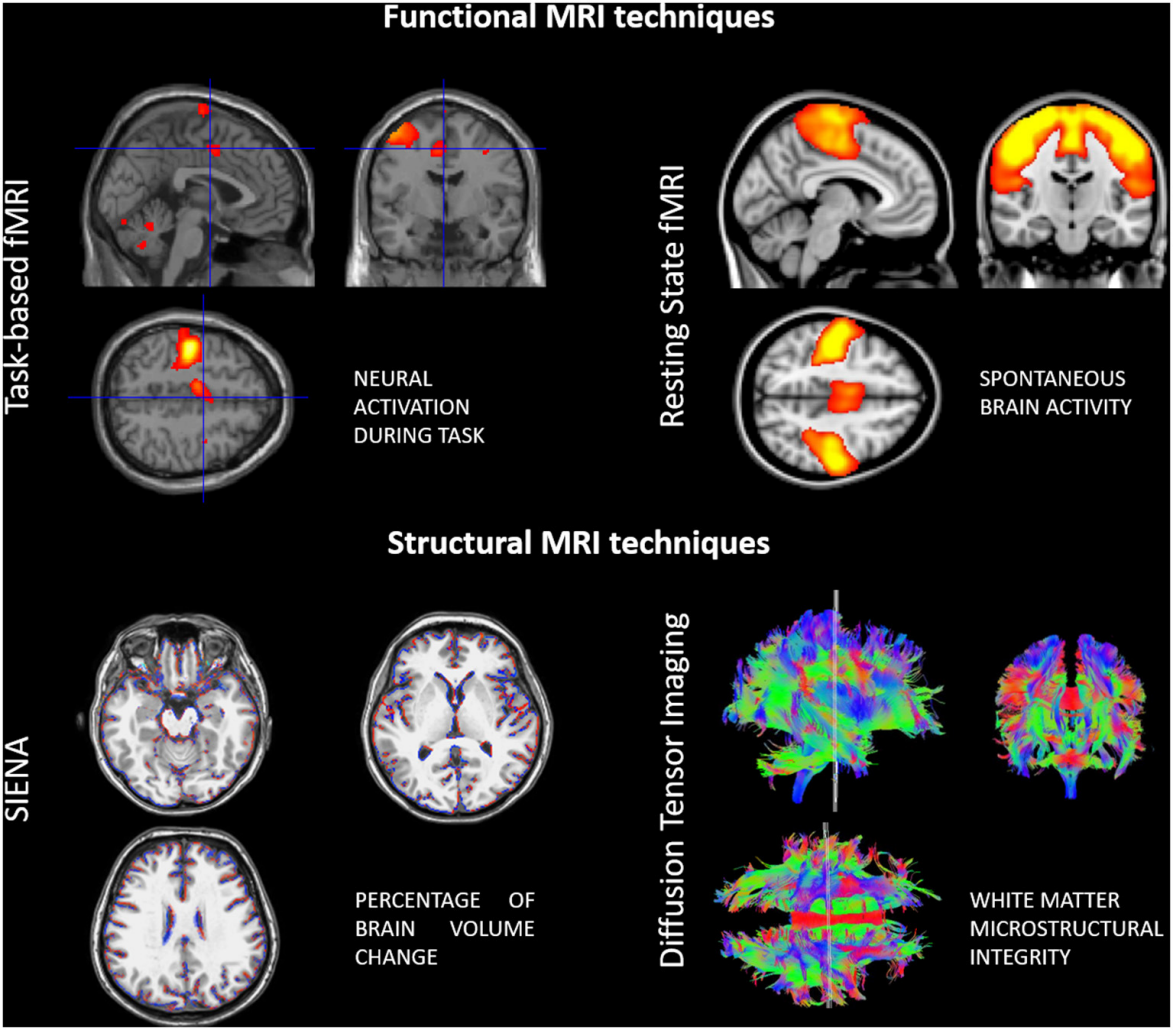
Examples of advanced functional and structural MRI techniques used for assessing neuroplasticity in multiple sclerosis patients.
